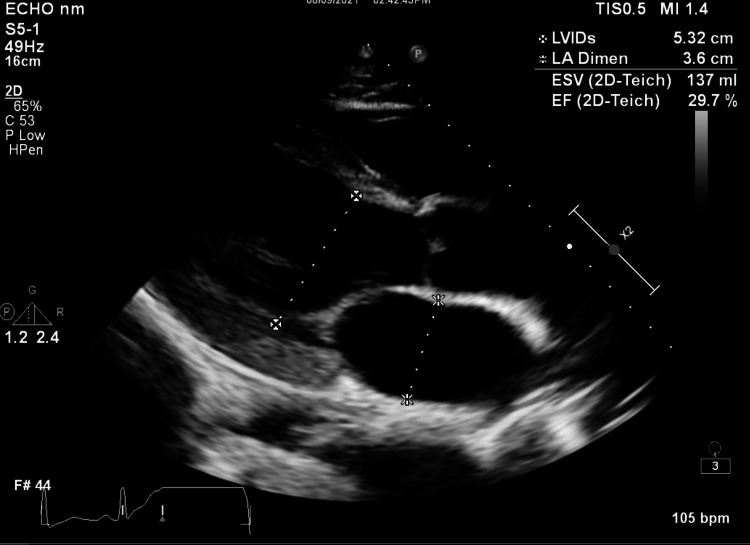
Transthoracic echocardiogram showing elevated systolic left ventricle internal dimension with left ventricular dilation
